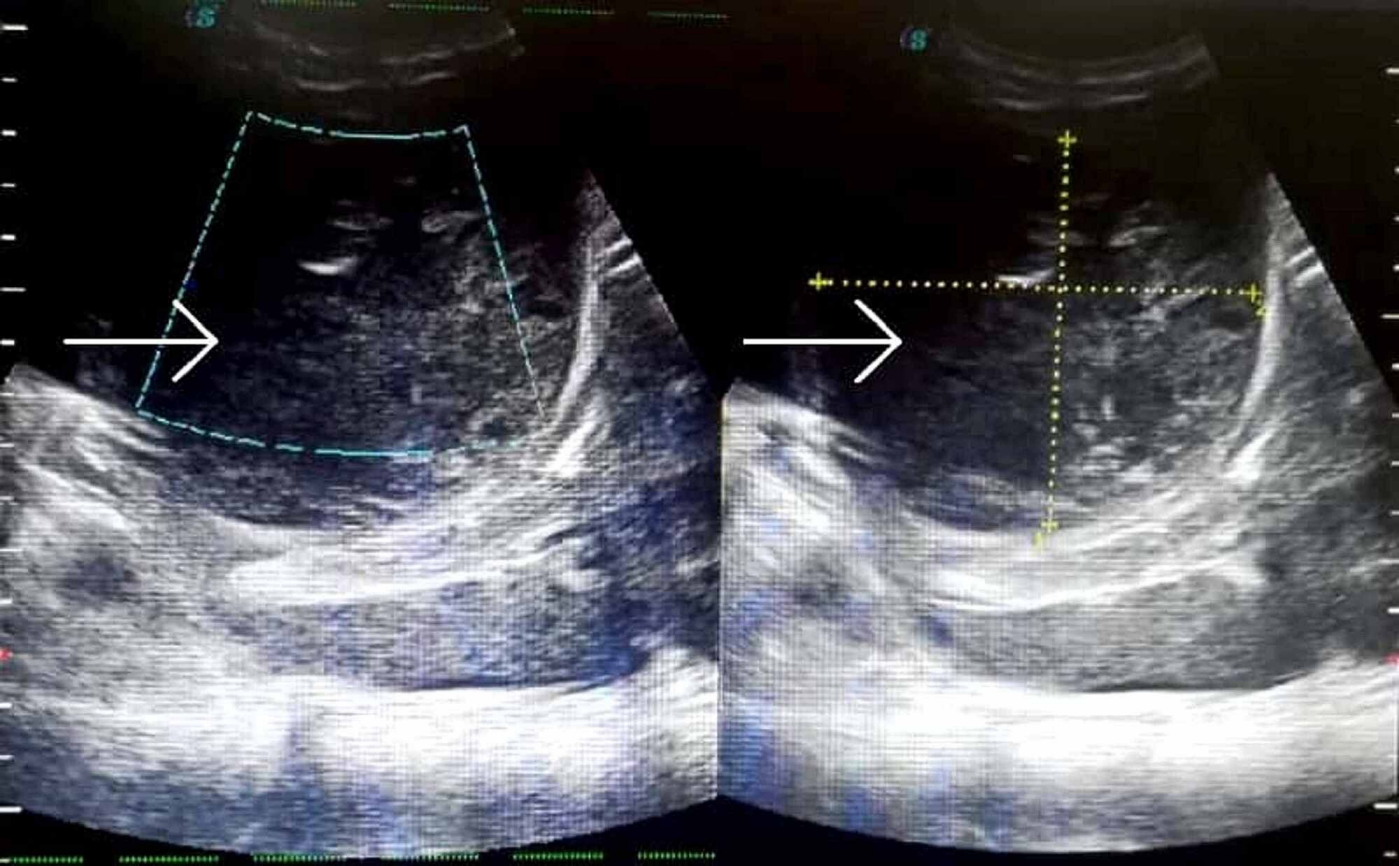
Abdominal ultrasound showing a large heterogeneous lesion, consistent with liver abscess, in the left lobe of the liver
